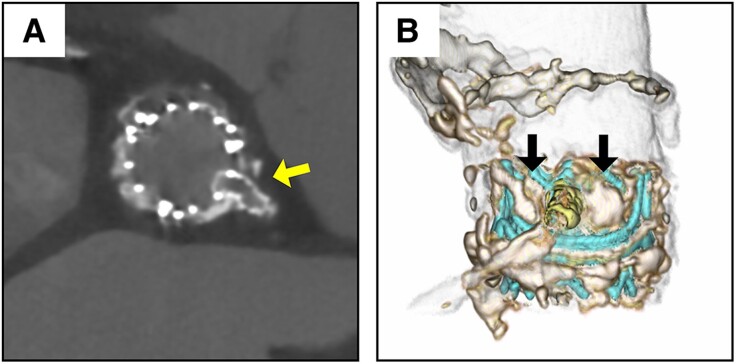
Post-procedural computed tomography. (*A*, *B*) Orthotopic stent (yellow arrow) and split leaflet (black arrow).
